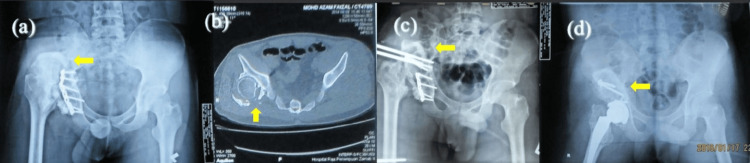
Post acetabular fracture dislocation fixation complicated with acetabular defect, hip stiffness, and limb length discrepancy >7 cm These are the x-rays of a 23-year-old male. (a) Preop x-ray shows migration of proximal femur with osteoarthritis. Arrow showed acetabular defect with false acetabulum. (b) Axial CT scan shows migration proximal femur with acetabular defect (arrow). (c) First stage procedure by application of the monorail and autograft reconstruction of the acetabular defect. Arrow showed a femoral head used to reconstruct the acetabulum. (d) Second stage surgery: removal of monorail and total hip replacement procedure. Interval between first and second stage was 60 days. Arrow showed good osteointegration between the femoral head and the acetabulum defect.
